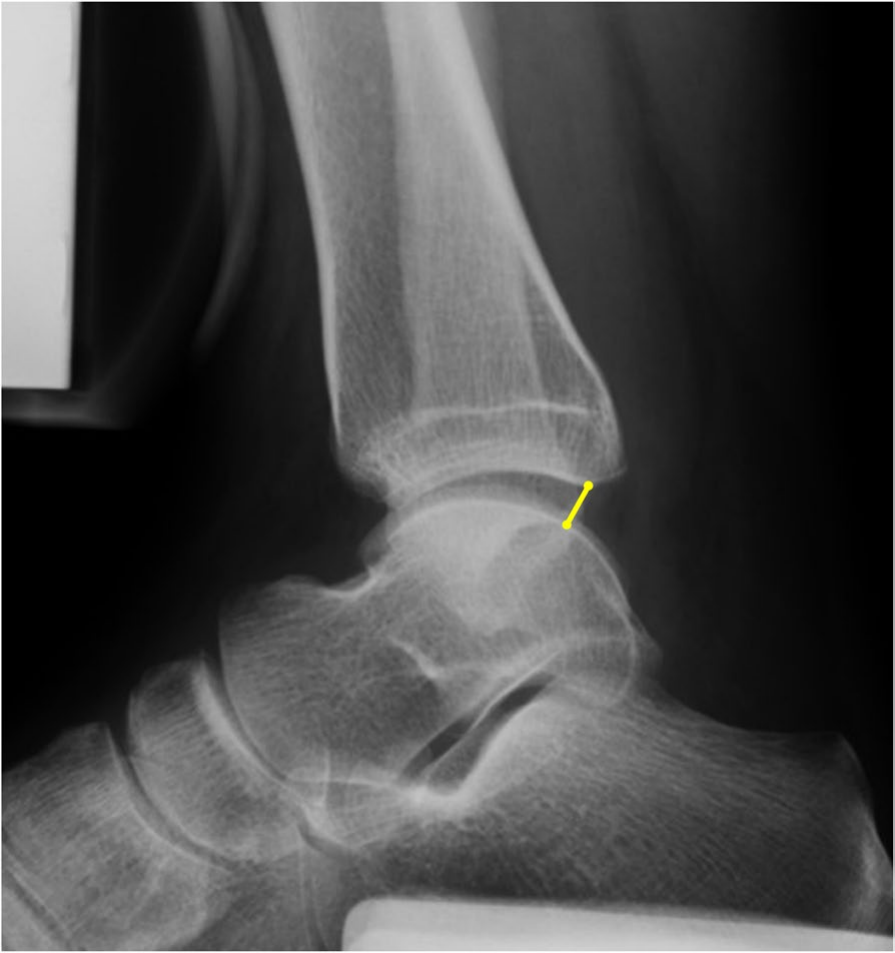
Radiographic measurement of anterior talar translation using TELOS stress device. Anterior talar translation is the distance between the posterior lip of the tibial articular surface and the nearest point of the talar articular surface (yellow line)
